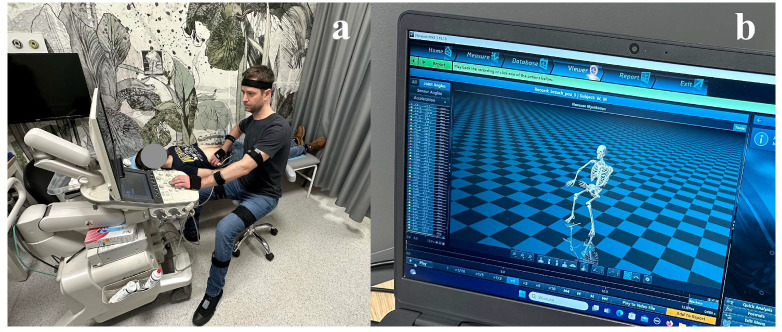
Kinematic evaluation during the testing phase: (a) motion sensors attached to the sonographer’s body and (b) software visualization of motion analysis in real time.
